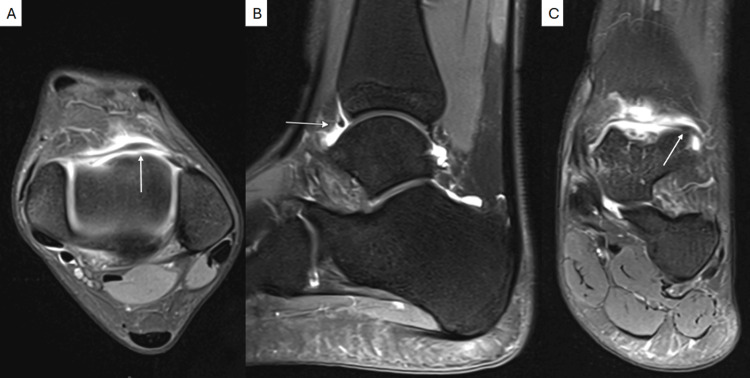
Axial (A), sagittal (B), and coronal (C) proton density fat-saturated MRI images. The intra-articular plica is denoted by the white arrows.
